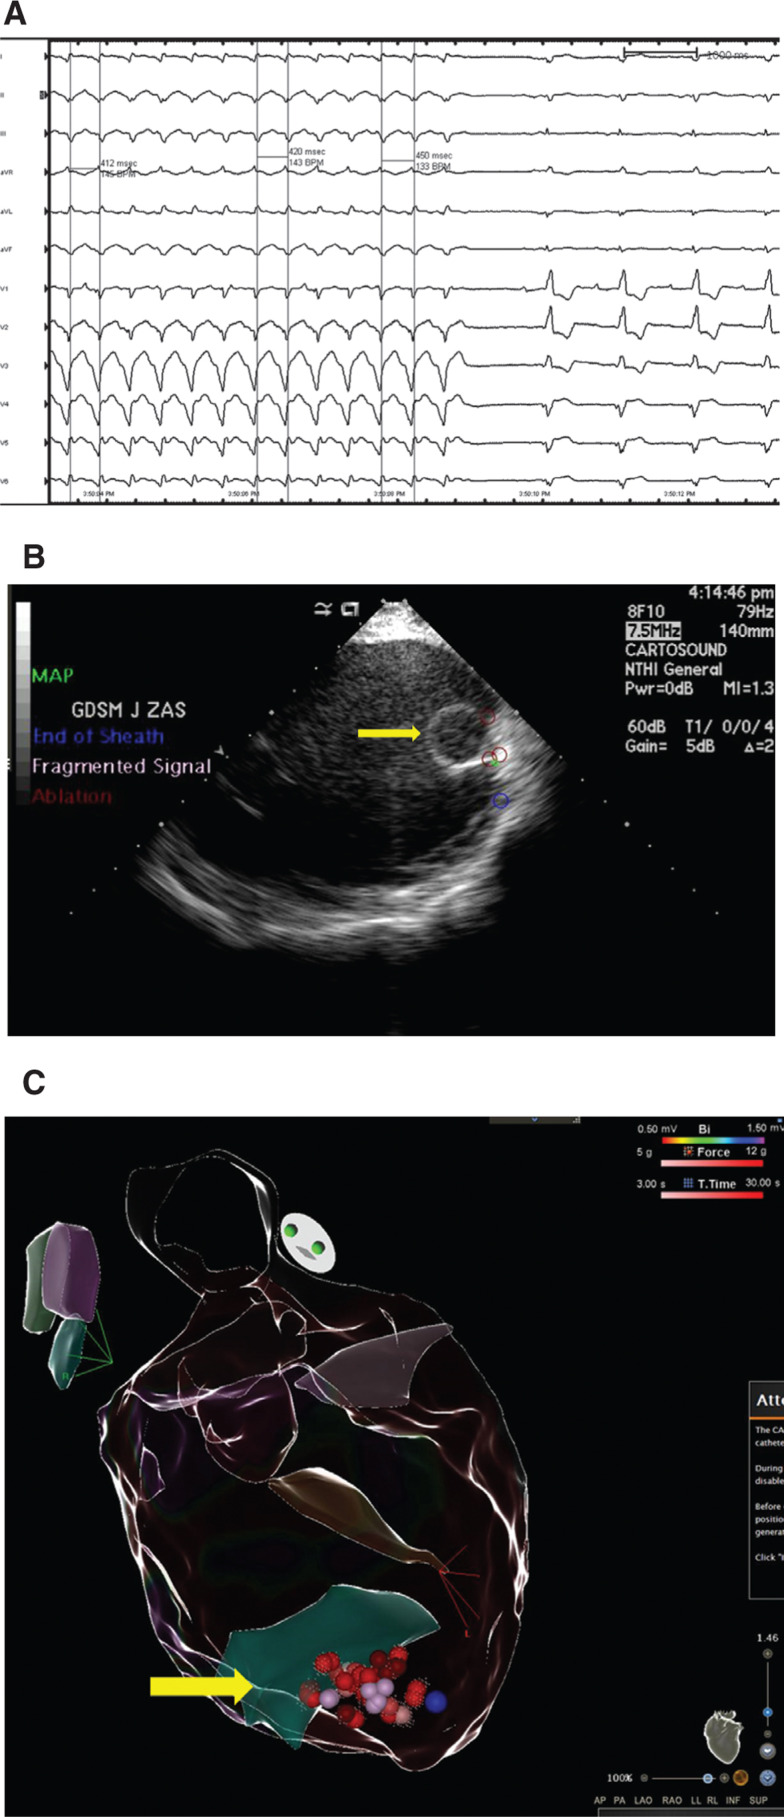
A: During ablation in the critical isthmus, the TCL was prolonged before termination. B: Intracardiac echocardiogram imaging shows the ablation lesions (red circles) near the LVAD cannula (yellow arrow). C: Electroanatomical map depicting the ablation lesions (red balls) near the LVAD inflow cannula (yellow arrow).
